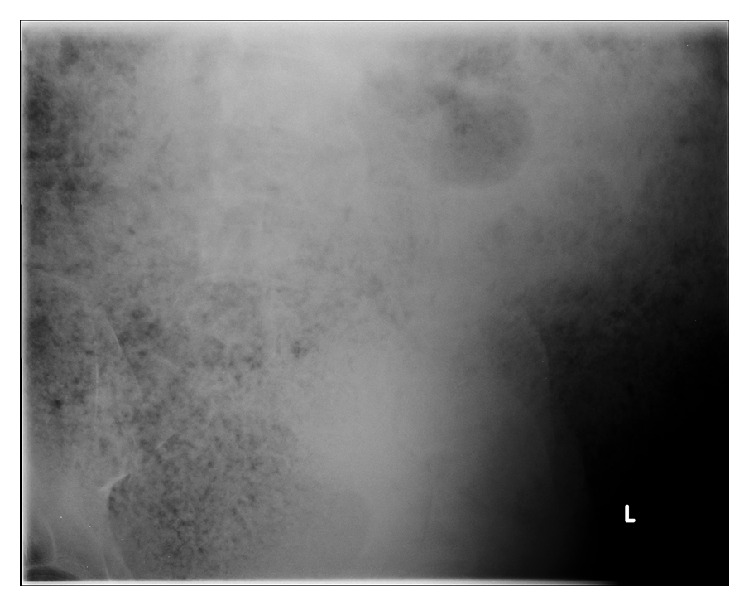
Plain preoperative abdominal radiograph.
